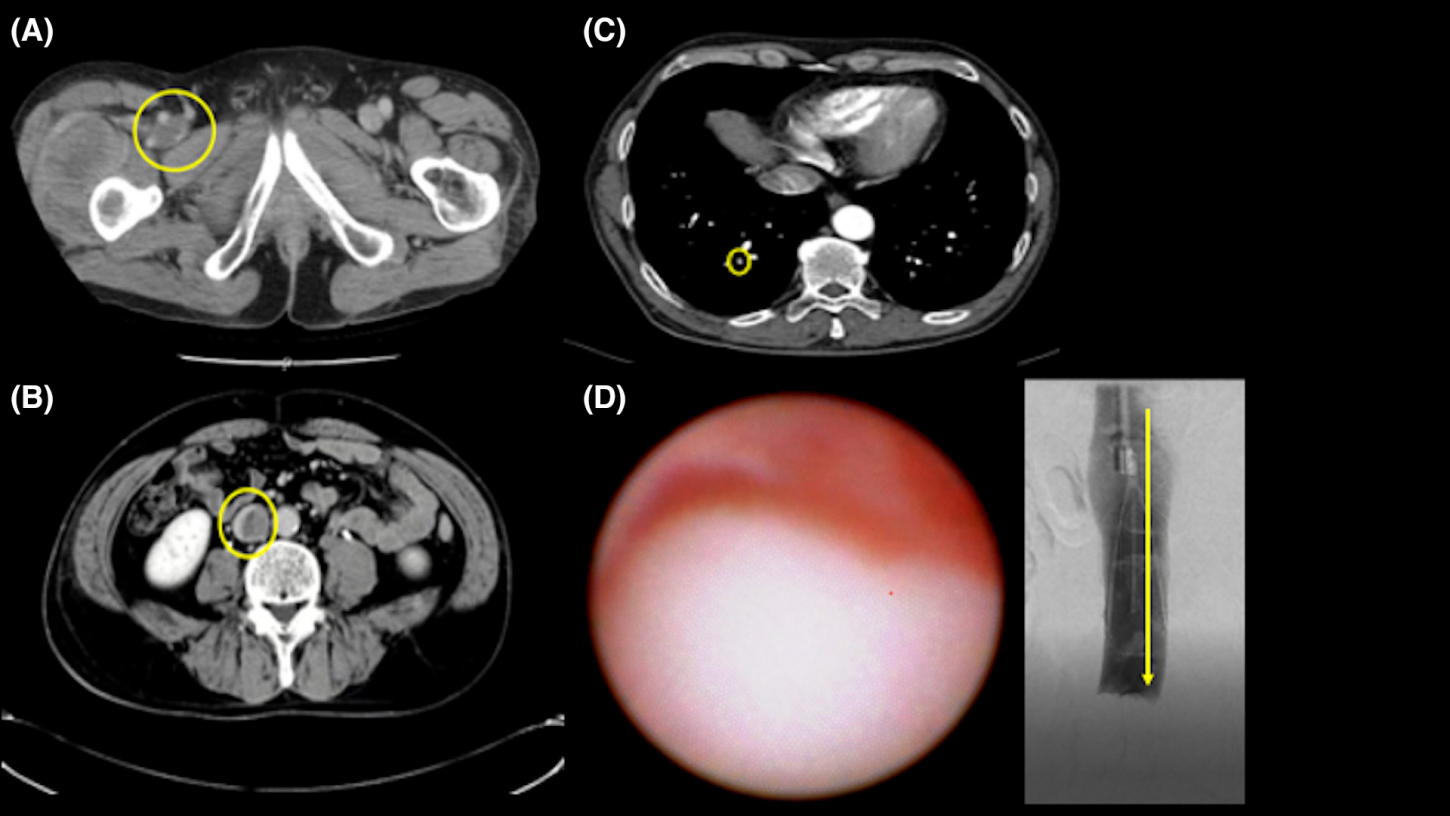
Contrast‐enhanced computed tomography showing images (A–C) and angioscopic image of the tumor thrombus (D). (A) A contrast defect image in the right femoral vein. (B) A contrast defect image in the inferior vena cava. (C) A new pulmonary embolism in right A10. (D) The surface was smooth with white solid components.
